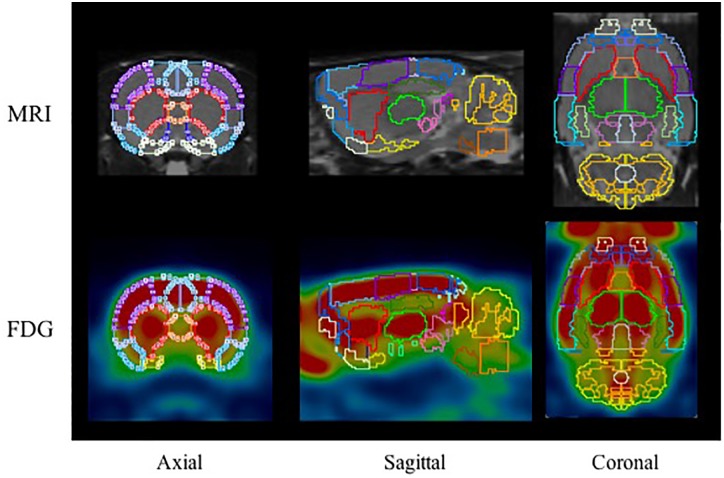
The ROIs applied to the PET images using MR imaging-based atlas by PMOD (top) and corresponding example for FDG (bottom). The main brain regions included: cerebellum (marigold), accumbens (blue), amygdala (yellow), striatum (red), hippocampus posterior (green), midbrain (purple), thalamus (lime), and cortex etc.
